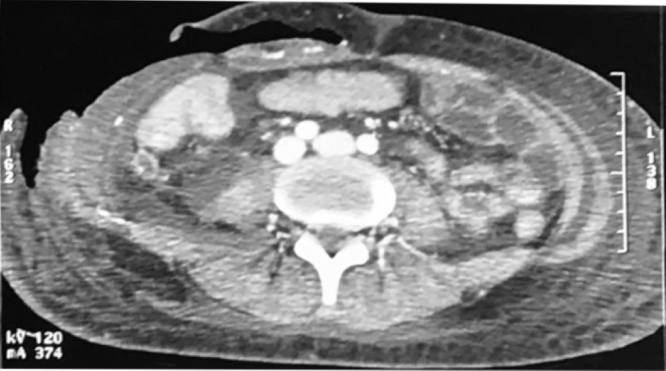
Post-operative axial section of a CT scan shows collections in the right iliac fossa in addition to peritoneal effusion.
